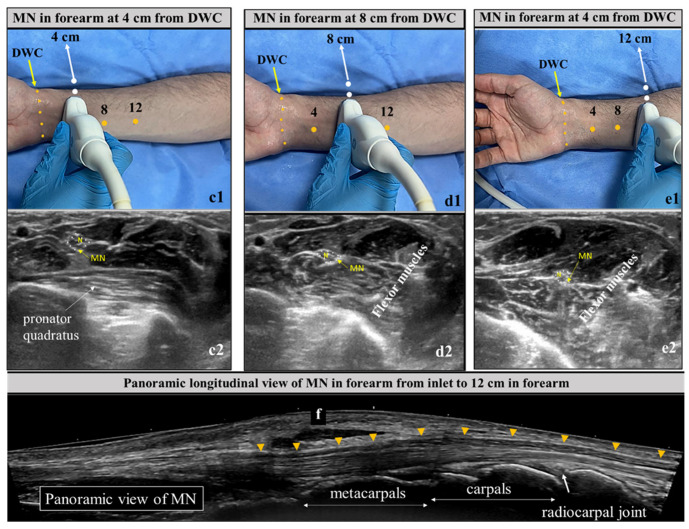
Pictures of wrist and forearm with reference markings of the sites at 4, 8 and 12 cm from DWC. Probe marker line (dotted white) is aligned with the markings to examine MN (c1,d1,e1). Corresponding transverse US appearance of MN at these points also shown. MN at 4 cm and 8, 12 cm are identified by pronator quadratus and flexor muscles, respectively (c2,d2,e2). Panoramic view longitudinal of the course of MN (arrowheads) from wrist to distal forearm also shown (f). *US = ultrasound, DWC = distal wrist crease, MN = median nerve.*
